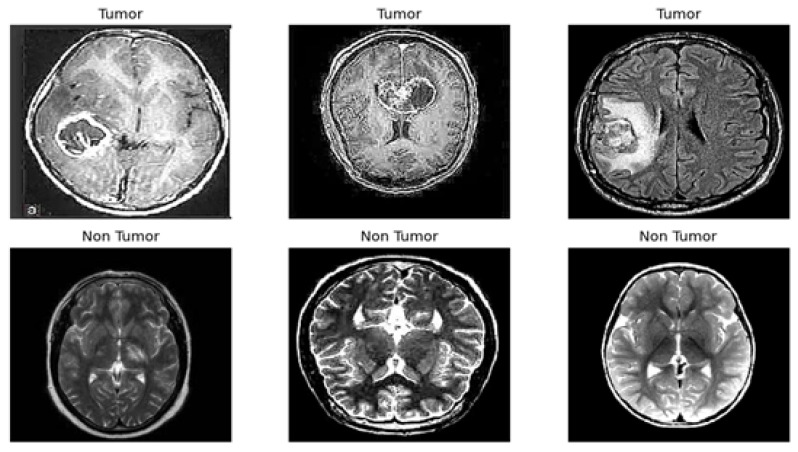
A visual representation of tumor and non-tumor MRI scans.
